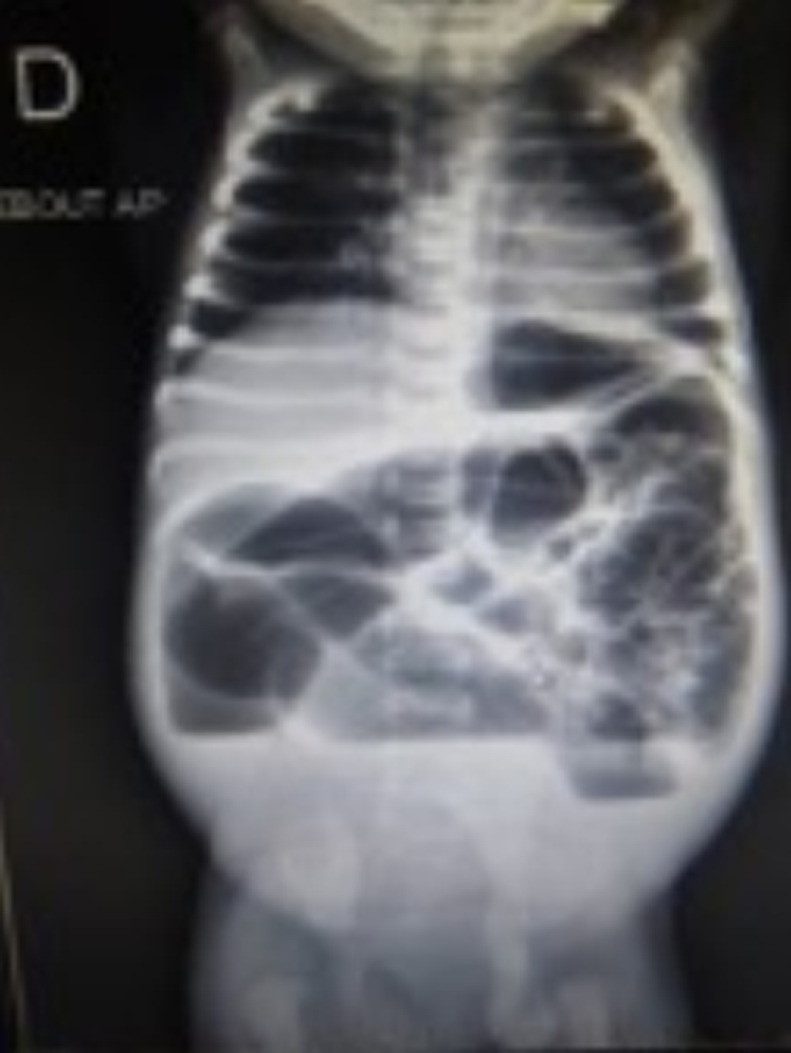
radiographie de l’abdomen sans préparation debout de face montrant une occlusion intestinale avec une absence d’aération du rectum
